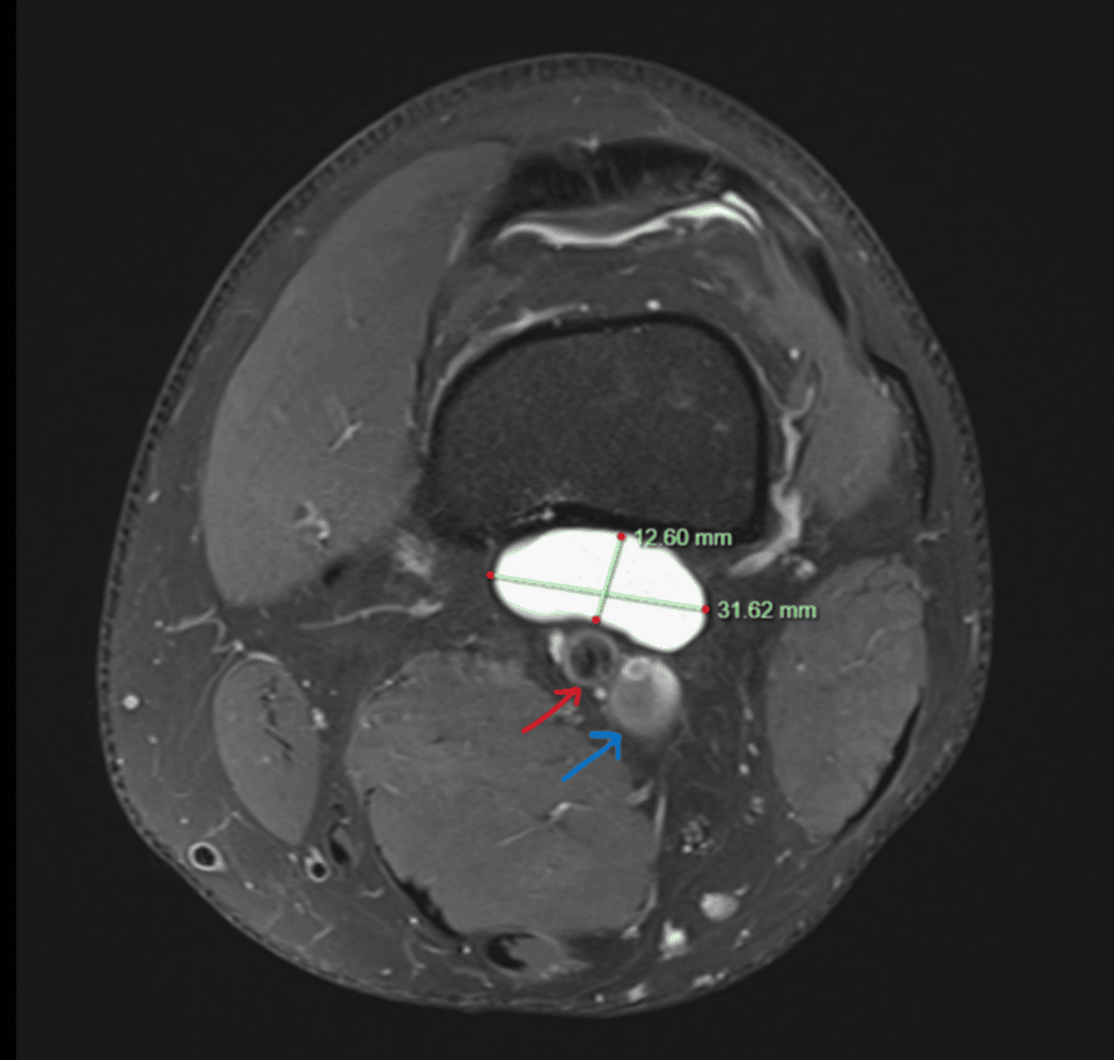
T2-weighted MRI of the left knee (axial view) shows a well-defined, ovoid cystic structure measuring 31.62 × 12.60 mm, abutting the posterior cortex of the distal femur and positioned anterior to the popliteal artery (red arrow) and popliteal vein (blue arrow).
